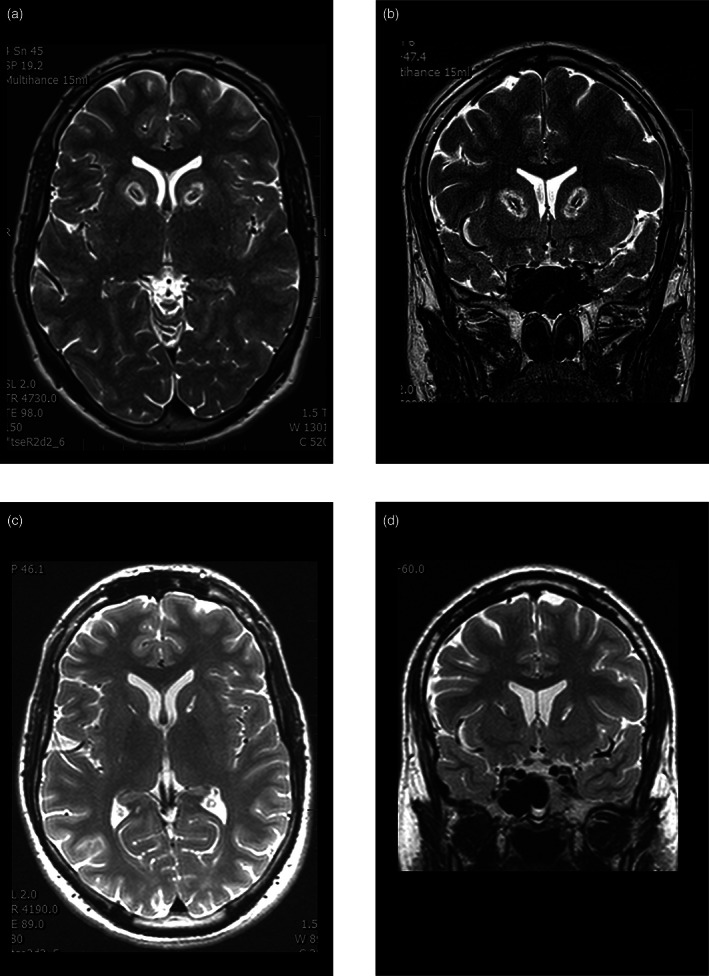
Magnetic resonance imaging examination, 1 day after thermolesion. Structural changes consisting of three coagulations in the right and left anterior limbs of the internal capsule. (a) Axial T2 SE image. (b) Coronal T2 SE image. Magnetic resonance imaging examination, 6 months after thermolesion. Reduced structural changes consisting of three coagulations in the right and left anterior limbs of the internal capsule. (c) Axial T2 SE image. (d) Coronal T2 SE image.
